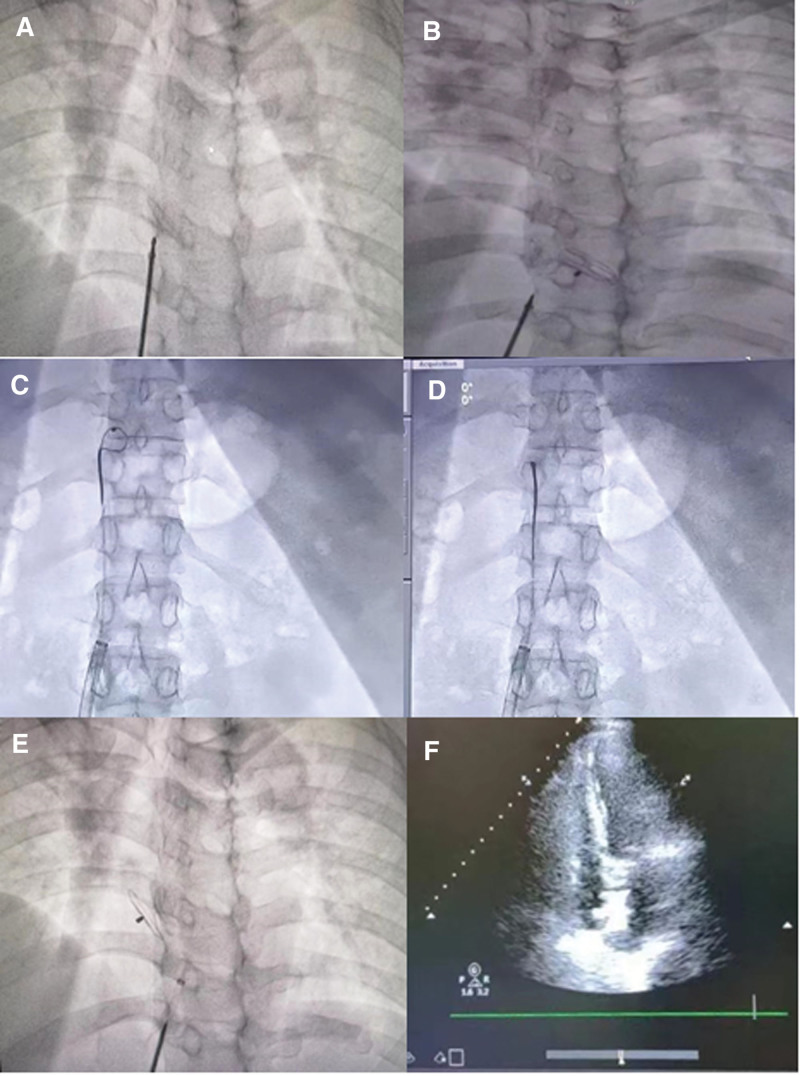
Closure, detachment, and interventional removal of the occlusion device during the patent foramen ovale. (A) The image before the occlusion device release. (B) The occlusion device detached after release. (C and D) The snare captured the single rivet end of the occlusion. (E) Successful closure of patent foramen ovale after interventional treatment. (F) The cardiac ultrasound revealed that the occlusion device was well-positioned.
